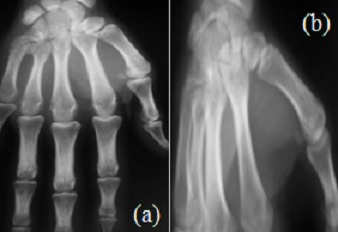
Radiographie de la main droite (A: incidence de face, B: incidence oblique) fracture-luxation dorso-latérale négligée de la base du M5
